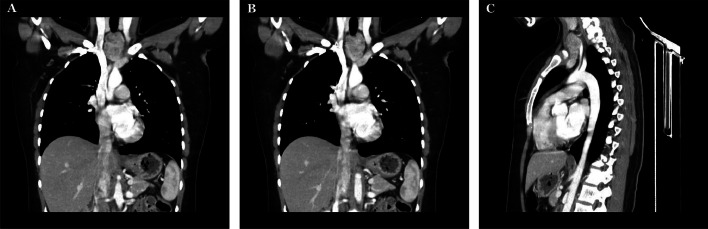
A coronal chest CT showing a retrosternal mass that is separated from the thyroid gland by fatty tissue and is attached to the great vessels (A and B). A sagittal chest CT reveals the mass’s retrosternal extensions and attachment to the superior vena cava (SVC) (C).
